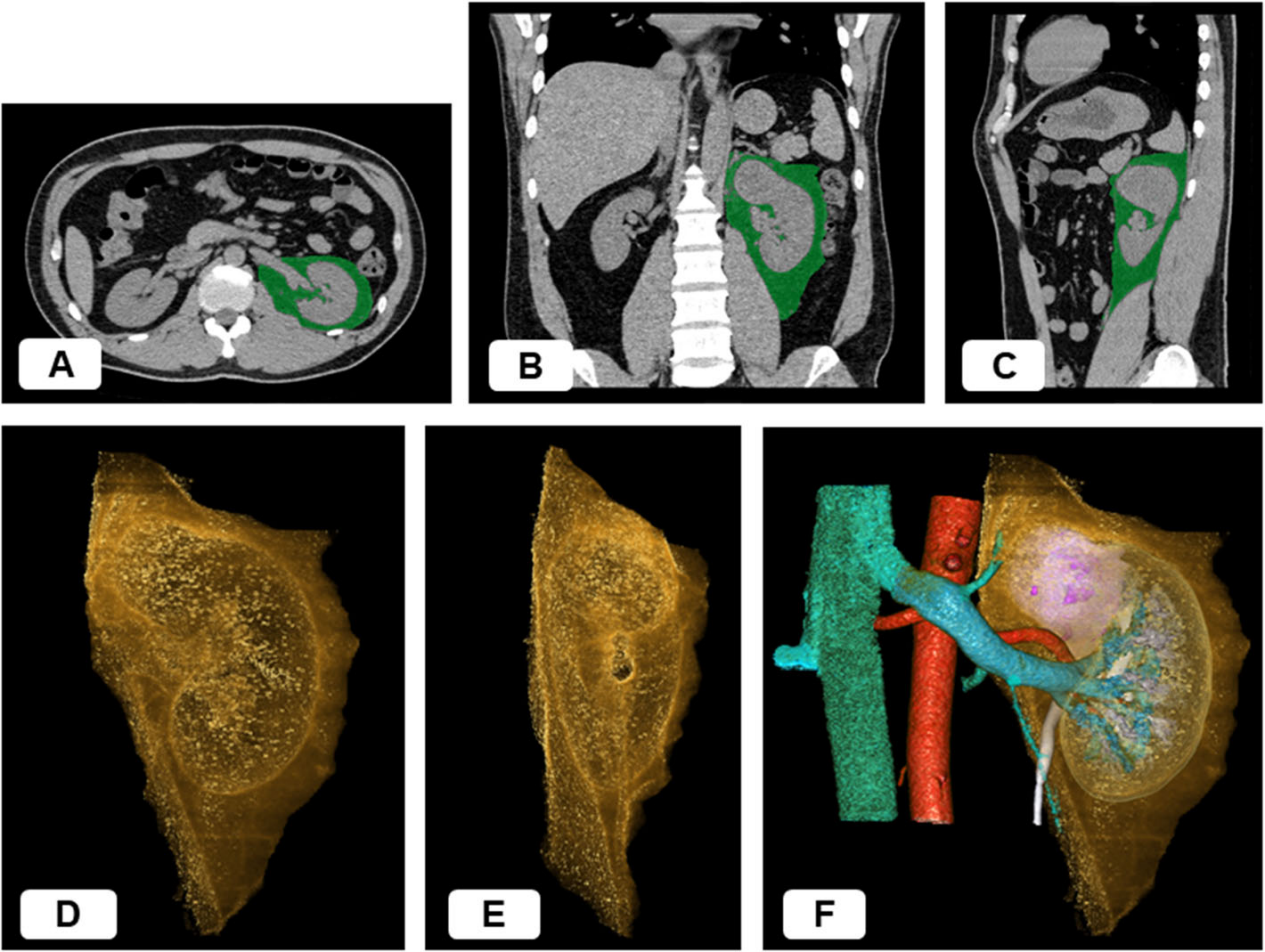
a, b, c: Preoperative non-contrast-enhanced computed tomography images for a patient with a localized renal mass located at the upper pole of the left kidney. The green area shows the region of interest corresponding to the perinephric fat defined as the fatty area within the anatomical structures, including the lateroconal fascia, fusion fascia, psoas muscle, lumbar quadrate muscle and diaphragm. a, Axial section. b, Coronal section. c, Sagittal section. d, e, f: The three-dimensional (3D) images for the perinephric fat reconstructed from the digital imaging and communications in medicine data according to the region of interest. The volume of this 3D structure was then calculated quantitatively. d, Anterior 3D view. The shape of the kidney and its upper tumor are stereoscopically excluded from the 3D structure. e, Inside 3D view. The lumens of renal hilar vessels are empty. f, Overlaid anterior 3D view showing the perinephric fat and other anatomical structures, including the kidney, artery, vein, ureter and tumor
